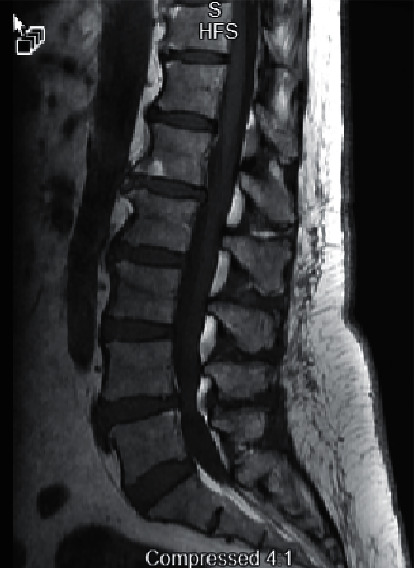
Lumbar MRI with contrast, demonstrating multilevel degenerative changes. There is facet arthropathy and ligamentum flavum hypertrophy. L4-5: there is a diffuse bulge slightly larger on the right. There is severe central canal stenosis. There is moderate right and mild-to-moderate left neural foraminal stenosis. L5-S1: there is a mild diffuse bulge slightly larger on the left with a tiny left foraminal annular tear. There is severe left and moderate right neural foraminal stenosis. L1-2: there is diffuse bulge and a superimposed central protrusion. There is mild-to-moderate central spinal canal stenosis.
